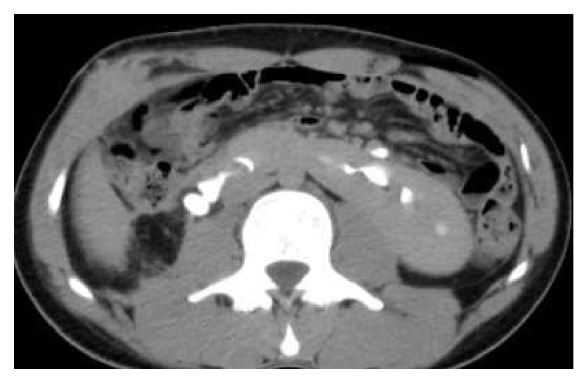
CE-CT following laparoscopic right nephrectomy showing leakage of contrast from the residual stump of the right lower pole.
